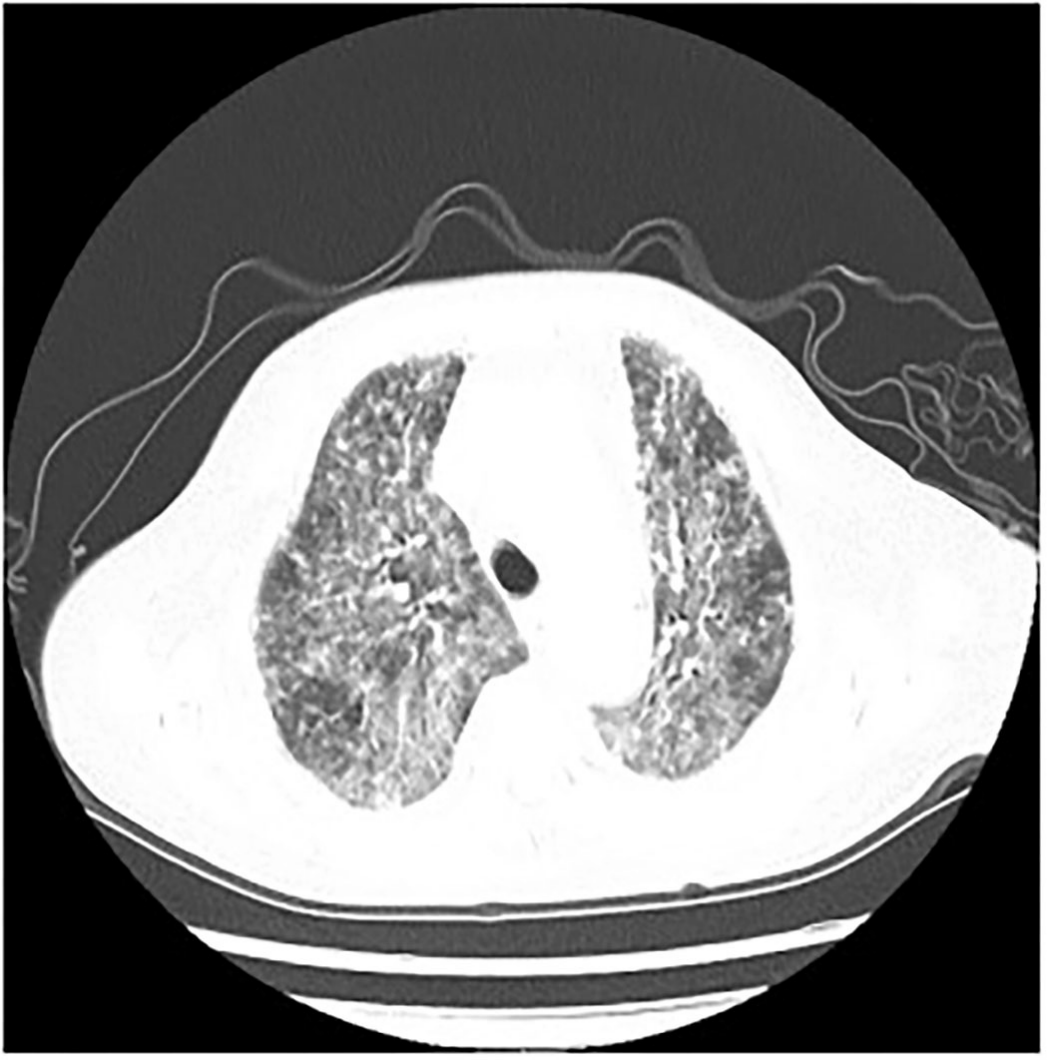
P. jirovecii* appearances on Chest CT scan in patient 2 as multiple patchy and flocculent high-density shadows in both lungs.
